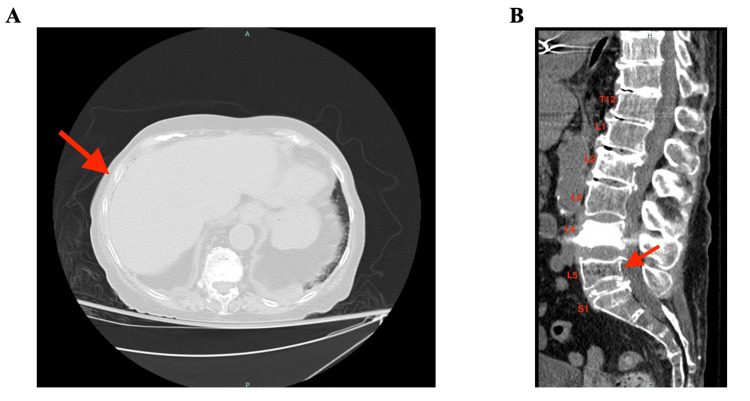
Fractures are a rare side effect of Mitapivat. A 75-year-old female with hereditary hemolytic anemia due to pyruvate kinase deficiency developed a rib and vertebral body fracture 18 days after starting Mitapivat. A: CT chest demonstrating nondisplaced fracture at the lateral right ninth rib. B: CT spine demonstrating multiple compression fractures, including L2, L4, and L5 vertebrae. L2 and L4 findings are consistent with chronic fractures with known kyphoplasty at L4. Radiological findings of L5 are consistent with a new compression fracture.
